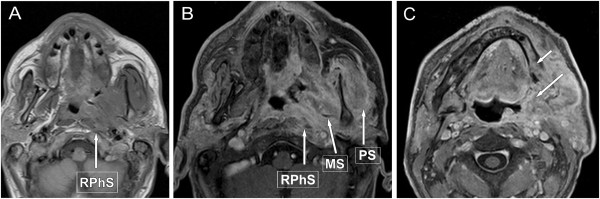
Oropharyngeal cancer, stage T4b. A: axial, non-enhanced T1-weighted, B, C: axial post-contrast T1-weighted MR images with fat suppression show tumour involving the lateral wall of the oropharynx on the left side, widely extending towards the retromolar trigone of mandibule (C image, long arrow), destructing the mandibular cortex, involving the marrow space (C image, short arrow). Note the extensive tumor involvement (long arrows) of the retroharyngeal- (RPhS), carotid-, masticator- (MS), as well as the parotid space (PS).
